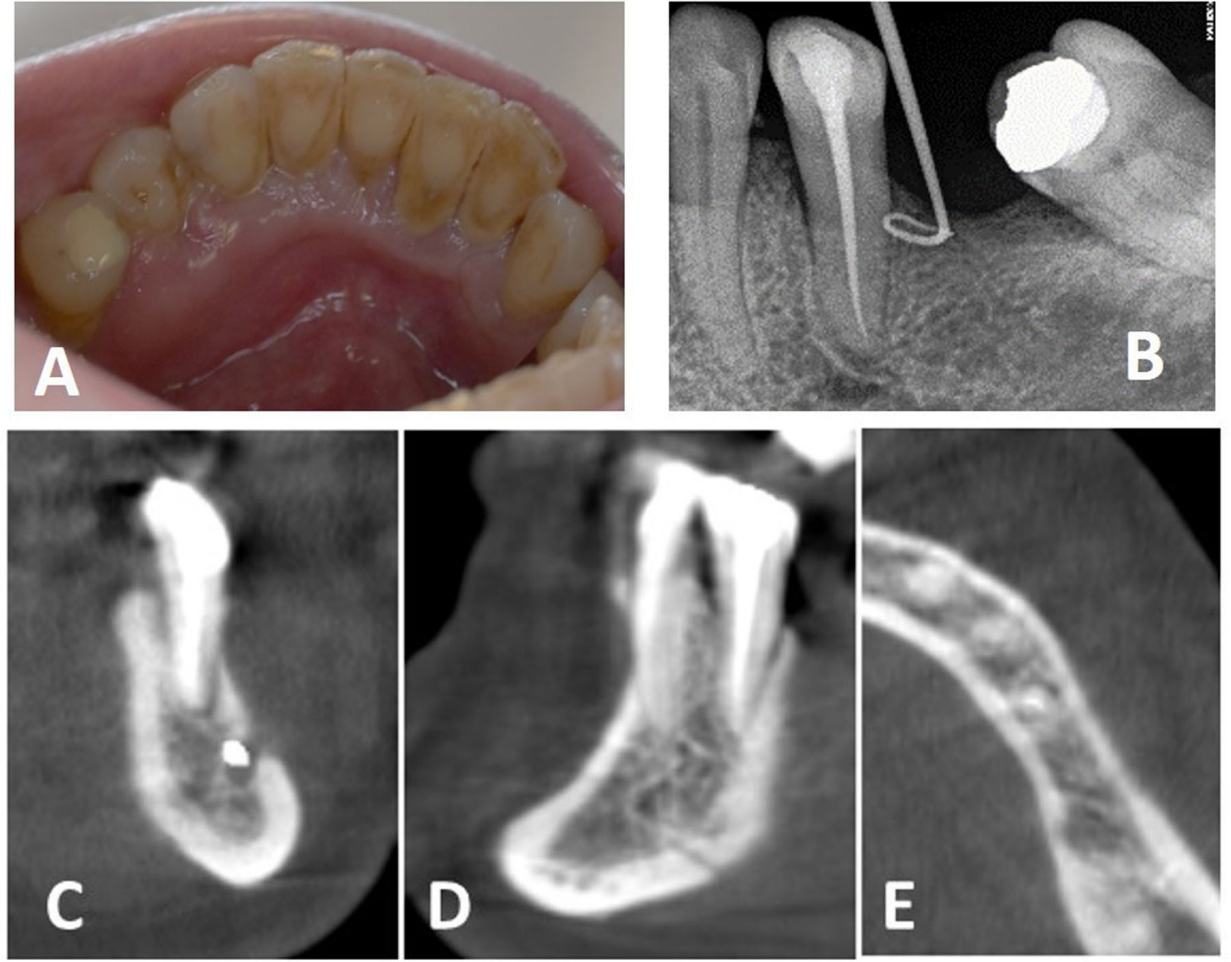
(A) Lingual tumefaction facing the lower left second premolar. Calculus is visible in the lingual part of the mandibular incisors. (B) X‐ray with a gutta‐percha cone inserted into the sinus tract identified the edentulous crest as the source of the pus. A PAI score of 2 was estimated for the lower left second premolar. (C–E) Frontal, sagittal and horizontal CBCT slices. A widening of the periodontal ligament is visible with no obvious changes in the structure and density of the alveolar bone.
